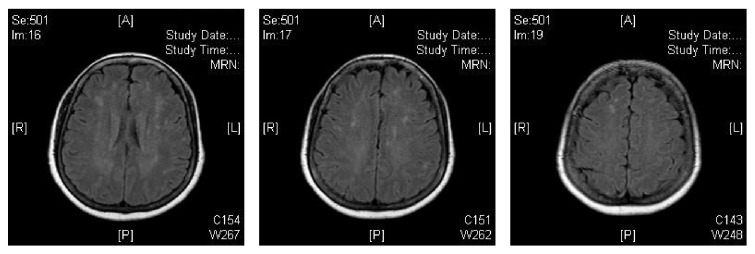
The patient's MRI performed two weeks later, which demonstrates white matter changes.
